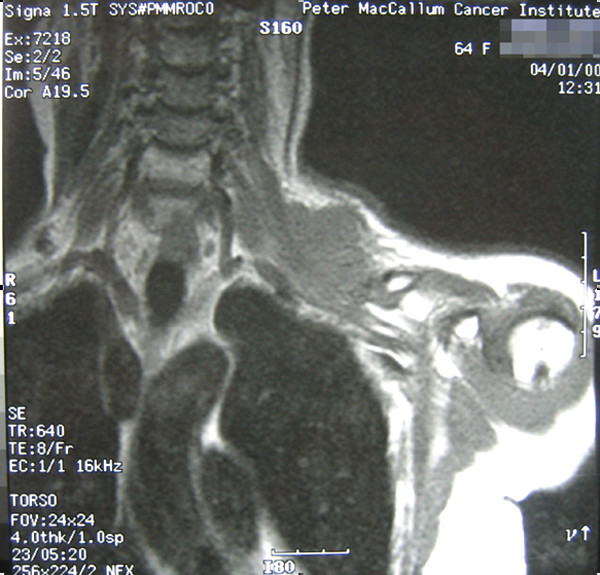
MRI showing soft tissue mass involving the upper branches of the brachial plexus.
